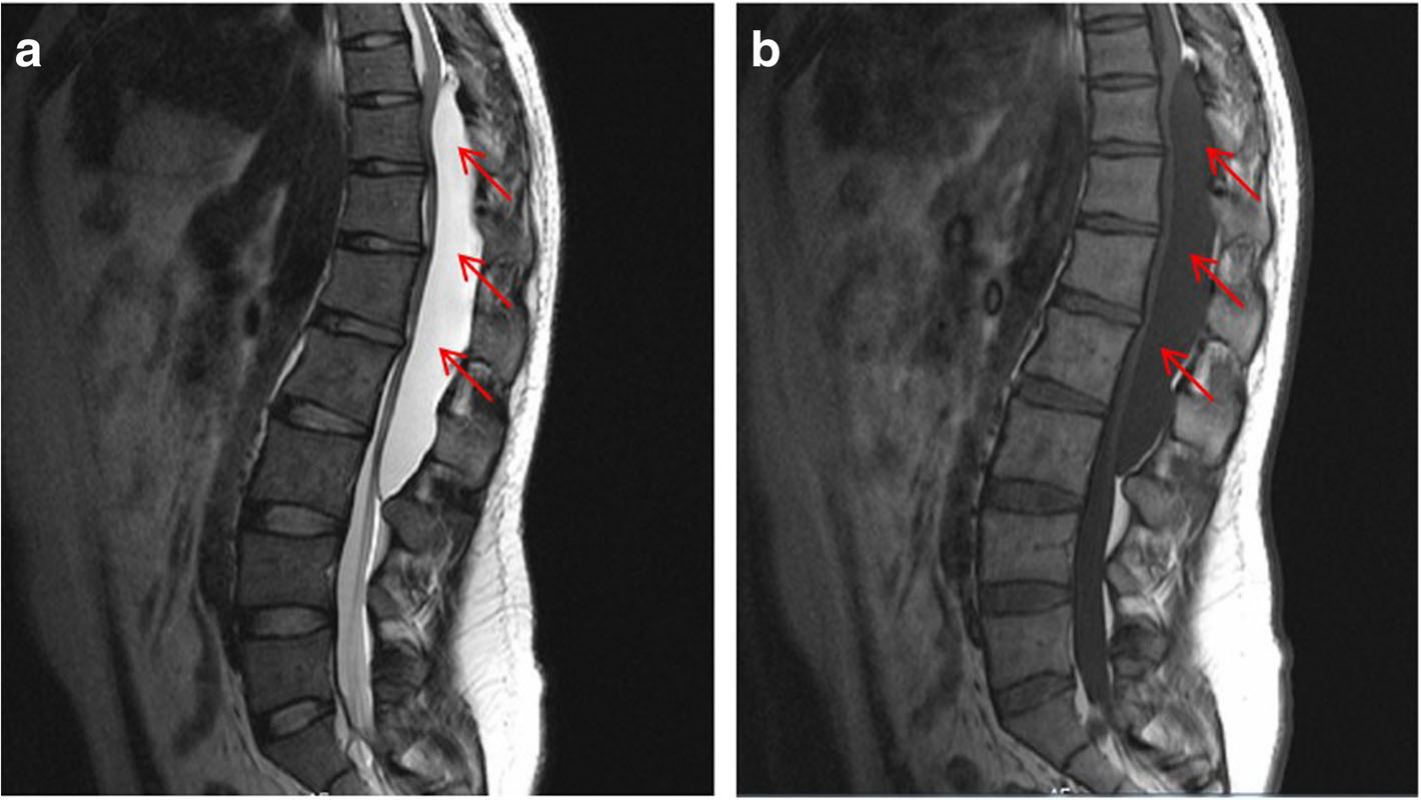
Magnetic resonance imaging revealed an intraspinal extradural tumor over T10 to L3 (red arrows), a Sagittal T2-weighted b T1-weighted
